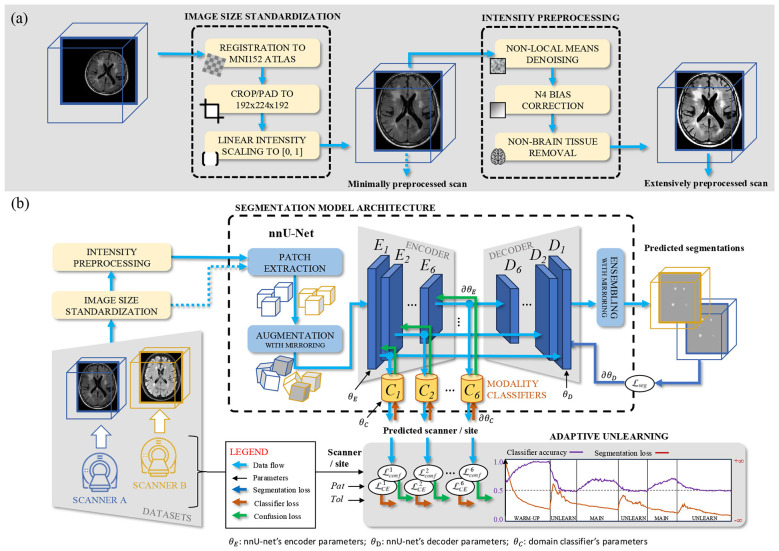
(a) MRI preprocessing and (b) adaptive multi-stage domain unlearning with nnU-Net.
